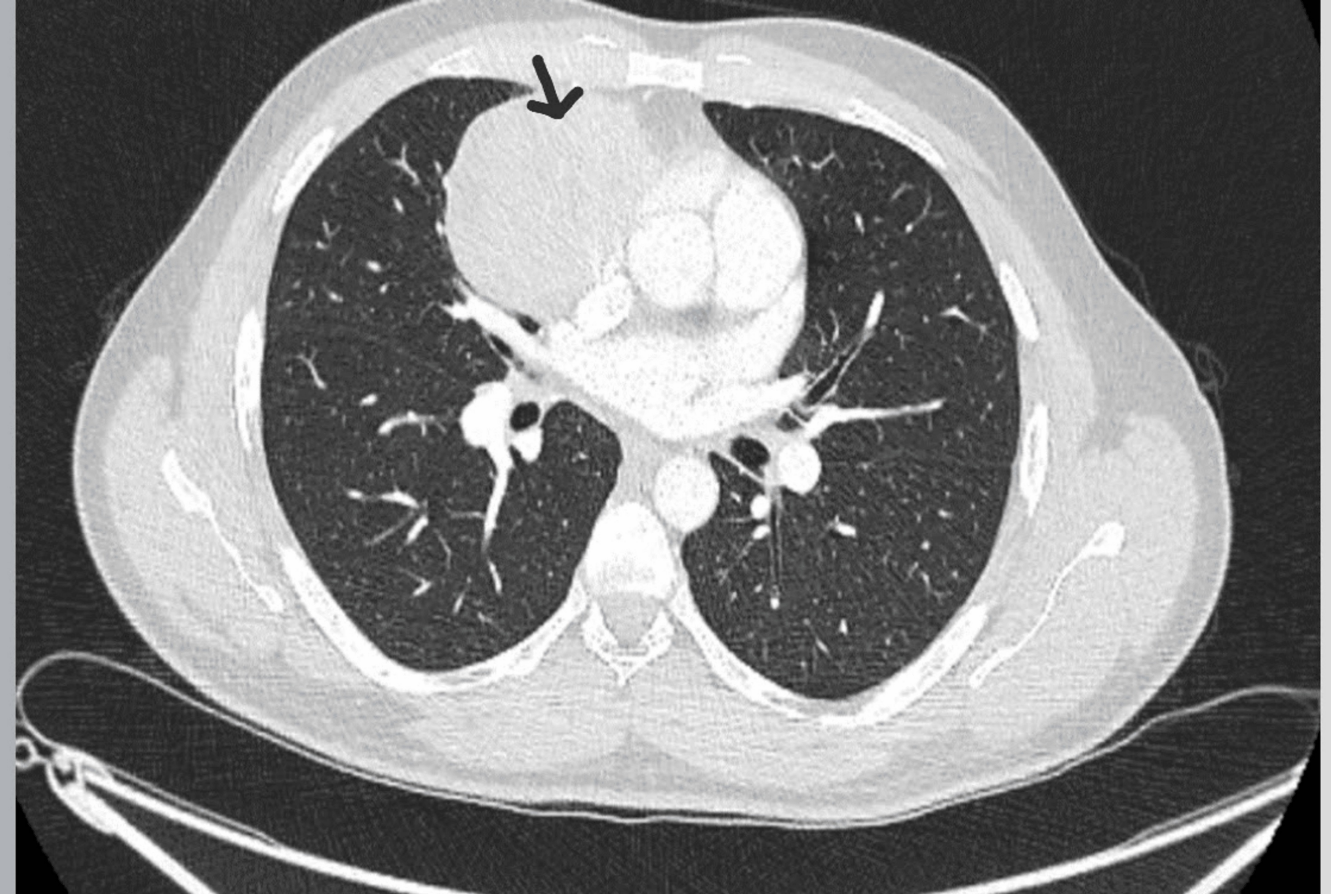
Chest CT conducted in 2022 showing a large anterior mediastinal mass.
